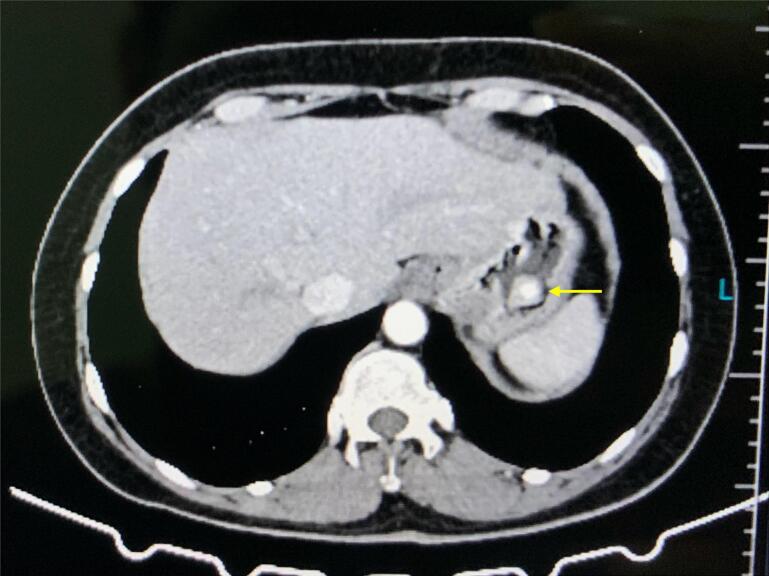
Abdominal CT with a solitary gastric lesion (arrow) and a hypervascular enhancement on arterial phase.
